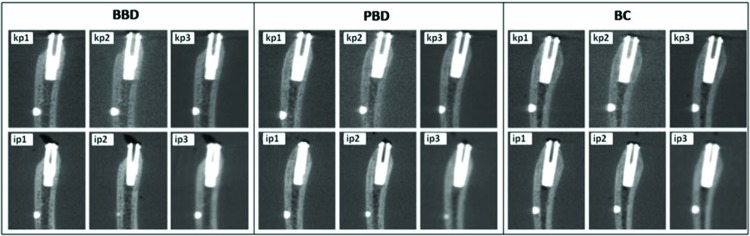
Image results from the BBD, PBD, and BC cases for the different image acquisition protocols from sagittal CBCT images.
